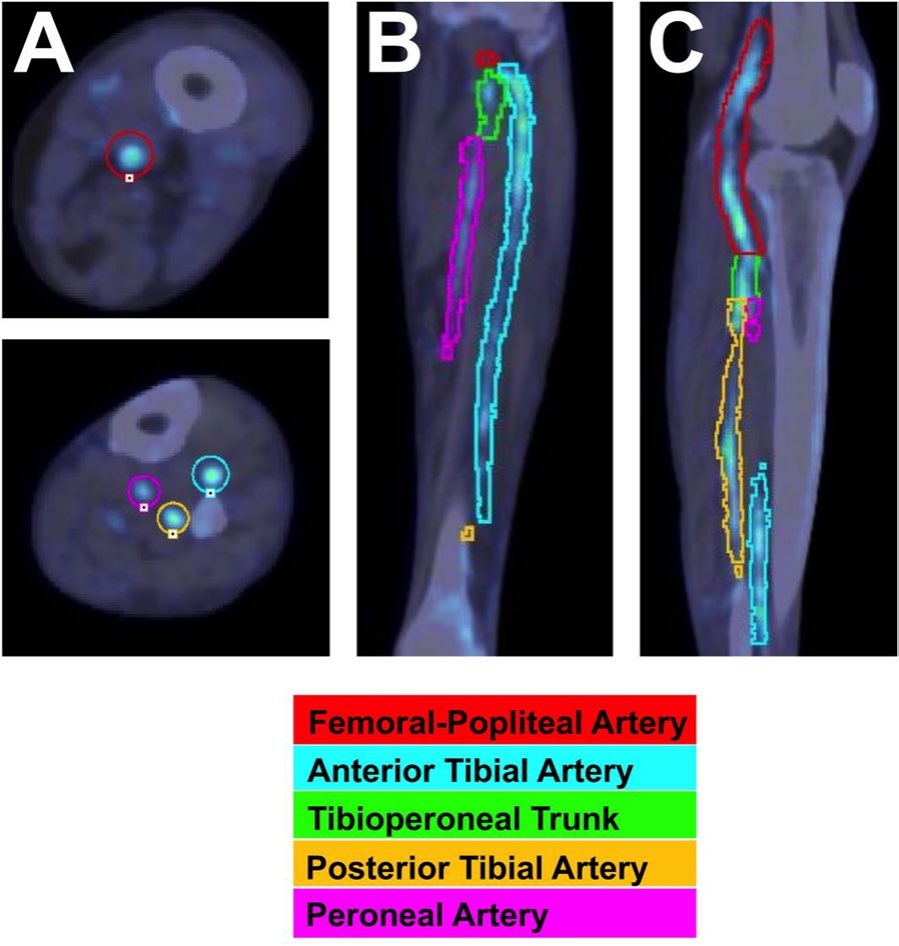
Representative example of lower extremity artery segmentation from CT images acquired in a patient with peripheral arterial disease. ROIs are displayed for arteries of interest in the thigh and calf and shown in A axial, B coronal, and C sagittal planes
